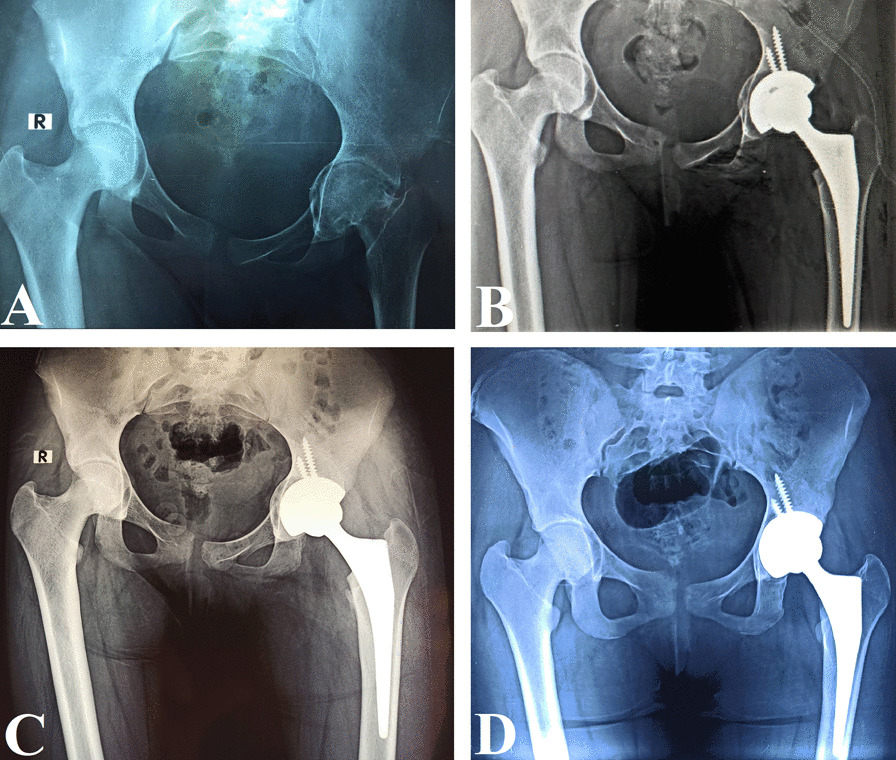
A 16-year-old female with protrusio acetabuli of the left hip treated with impaction bone grafting and primary ceramic on ceramic THA. A Preoperative pelvis AP X-ray. B Postoperative pelvis AP X-ray. C Six-month follow-up pelvis AP X-ray showing partial graft incorporation. D One-year follow-up pelvis AP X-ray showing full graft incorporation and remodeling
